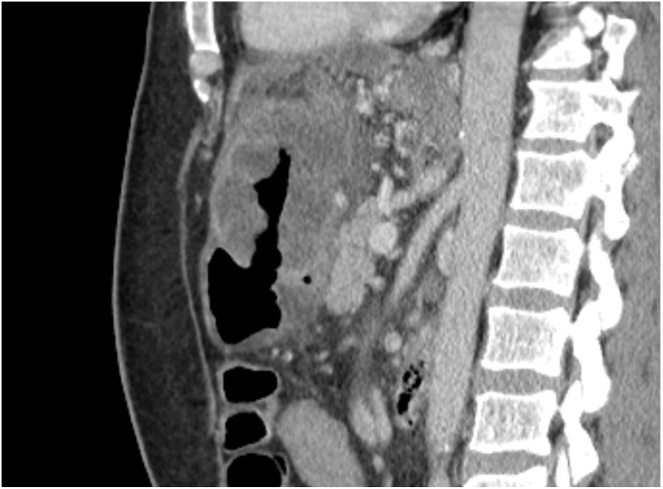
Sagittal CT sections demonstrate a necrotic left hepatic mass with fistulous communication with the gastric antrum.
